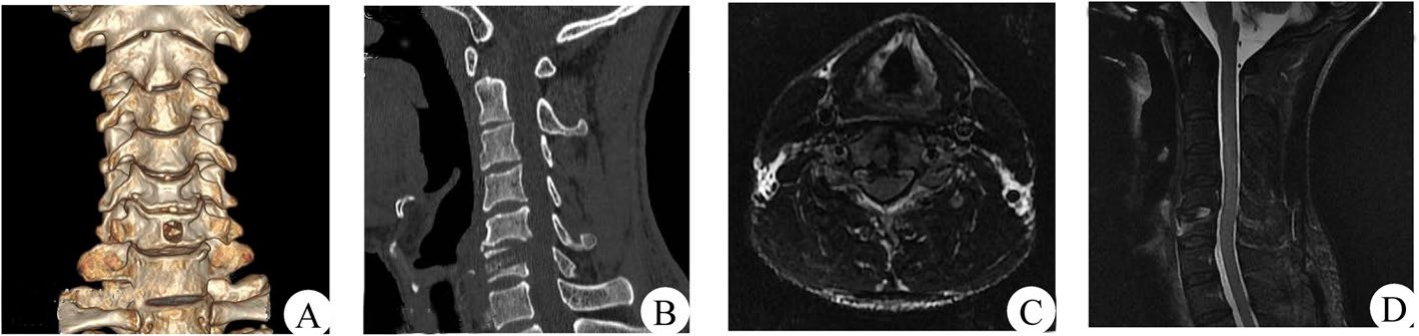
Ostoperative CT showed that the disc-bone complex was removed and 3D reconstruction showed intact vertebral tunnels with no loss of vertebral height and no fracture occurred (A, B). MRI showed adequate spinal cord decompression (C, D)
